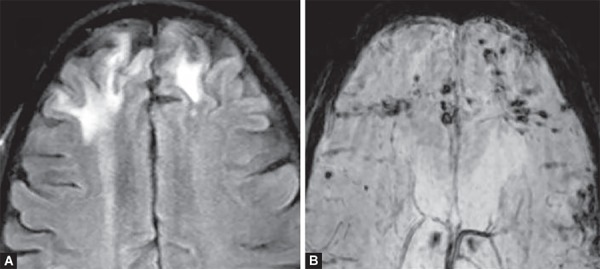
FLAIR hyperintense (A) areas of post traumatic contusions in bilateral frontal lobes, with multiple small hemorrhagic contusions picked up on SWI image (B) due to susceptibility blooming artifact secondary to hemorrhagic areas
